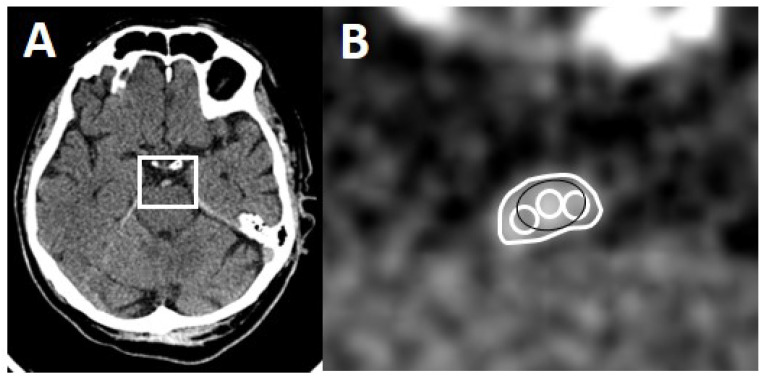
Methods of thrombus delineation. (A) Axial CT section with a hyperdense basilar artery. (B) Magnified region of interest (white box) with the three methods of thrombus delineation. Method 1: black RoI, Method 2: three white circles, Method 3: large white RoI.
